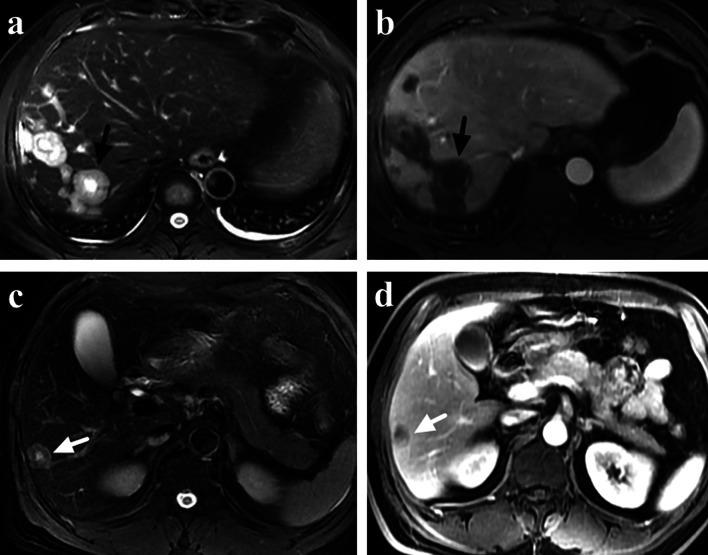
a, b A 40-year-old male HEH patient with coexistence of target appearance on both T2 weighted and portal phase images. a Target sign on fat-saturated T2-weighted image, consisted of concentric layers including markedly hyperintense in central tumor regions and moderately hyperintense peripheral zone (arrow). b Target sign on portal phase image, consisted of concentric layers including markedly hypointense in central tumor regions and peripheral zones which were moderately hypointense relative to liver parenchyma (arrow). c, d A 51-year-old male rectal carcinoma patients with multiple liver metastasis. c A classic target sign on fat-saturated T2-weighted image (arrow). d Heterogeneous enhancement on portal phase image (arrow), instead of target sign
